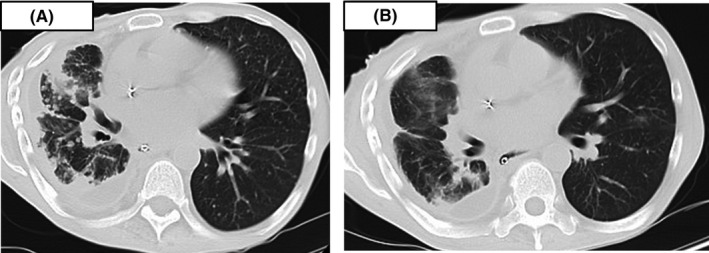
A, Chest computed tomography showing multiple lung metastases and pleural effusion. B, Chest computed tomography showing that the lung metastases disappeared
